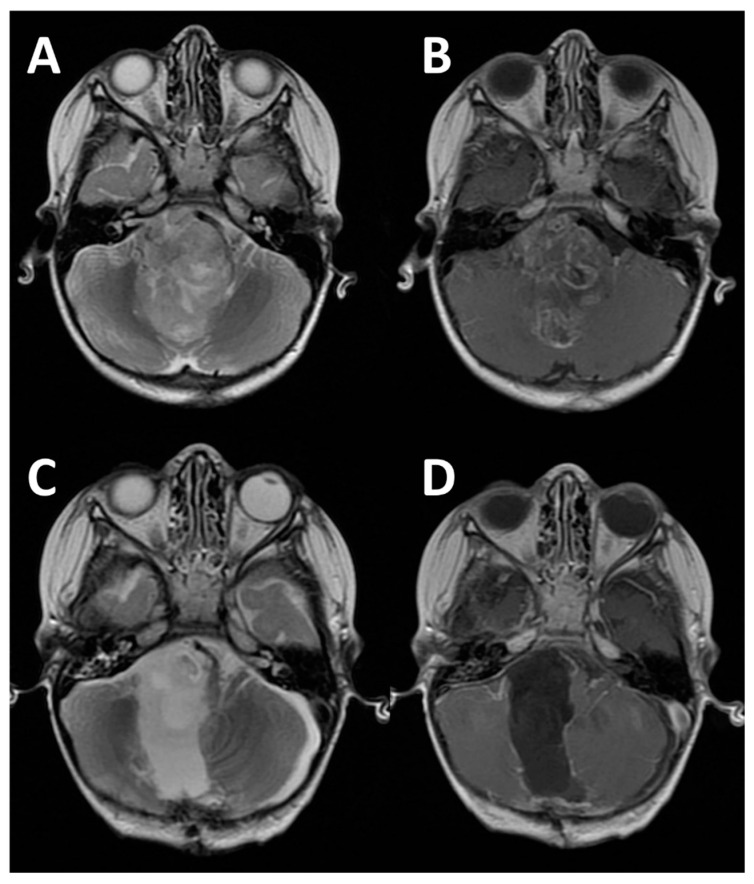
Axial T2 (A) and gadolinium MRI (B) showing of a 4-year-old boy showing a large PF-A ependymoma obstructing the IV ventricle, occupying large part of the posterior fossa and the right CPA. The same sequences (C,D) show a GTR after surgery (realized with exoscope).
